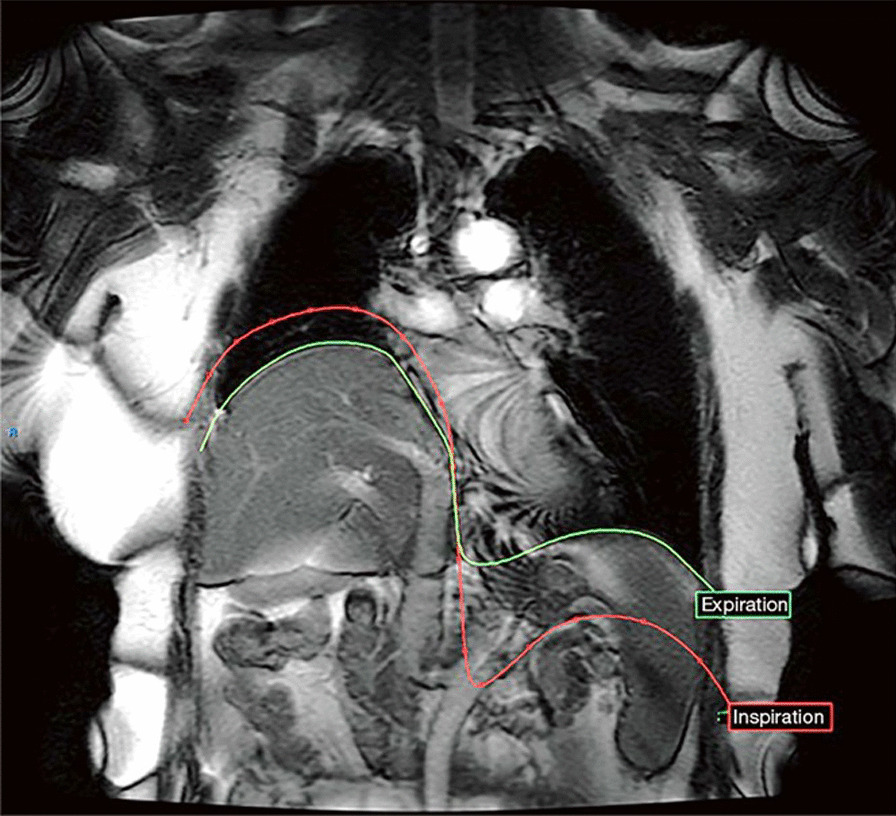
Coronal dynamic MRI of representative patient with right hemidiaphragm eventration due to hemidiaphragm paralysis. At the end of inspiration (red line), the healthy left diaphragm has descended caudally while the paralyzed right hemidiaphragm paradoxically moves cranially. At end exhalation (green line), the paralyzed right hemidiaphragm continues to be elevated relative to the left. Reproduced under Open Access Creative Commons License: Le Pimpec-Barthes et al. J Thorac Dis 2019;11(8):3467–3475
